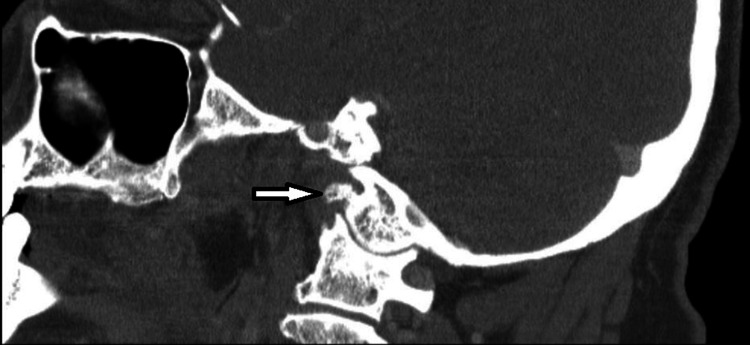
CT of the temporal bone sagittal section showing right AO joint osteophyte with extension into the hypoglossal canal. CT: computed tomography; AO: atlantooccipital
